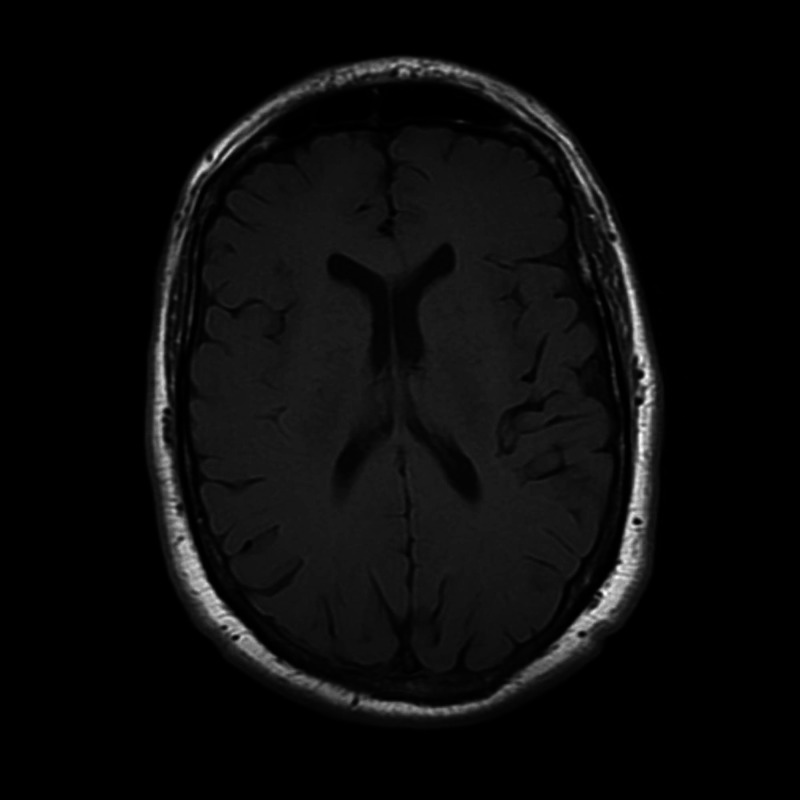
Follow-up non-contrast-enhanced MRI brain obtained one year following the initial event, with T1-weighted image showing absence of any deposits. MRI, magnetic resonance imaging
